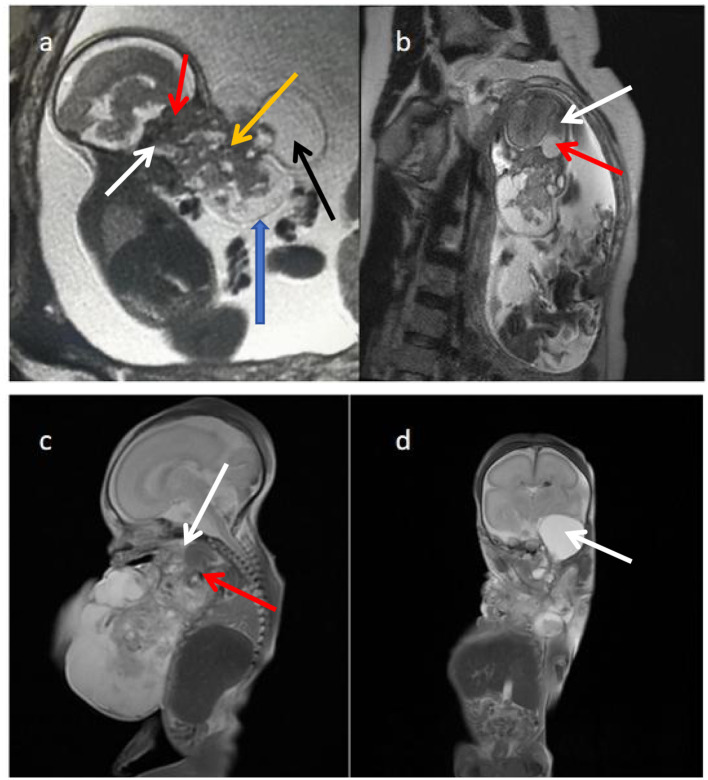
Prenatal and postnatal MRI findings of fetal oral immature teratoma with intracranial abnormality. (a) Prenatal sagittal T2-weighted MRI demonstrates a solid-cystic mass (blue arrows) measuring 6.1 × 6.1 × 6.8 cm arising from the oropharynx with broad attachment to the palate and skull base (red arrows). The solid component (S, orange arrows) shows intermediate signal intensity, while the cystic component (C, black arrows) exhibits markedly high T2 signal. The mass causes severe oropharyngeal compression (white arrows), correlating with the risk of upper airway obstruction. (b) Prenatal coronal T2-weighted MRI reveals a well-defined hyperintense lesion (red arrows) in the right temporal lobe, measuring 17 × 17 mm, compatible with an arachnoid cyst. Mass effect on the adjacent temporal lobe is evident (white arrows). The coexistence of an intracranial cystic lesion with an oral teratoma raises consideration of a developmental field defect involving midline structures. (c) Postnatal sagittal T2-weighted MRI confirms the broad-based attachment of the residual mass to the skull base and mandible (white arrows), with associated bony erosion (red arrows). (d) Postnatal coronal MRI shows a left temporal meningocele (arrow), an additional intracranial finding that further supports the presence of concurrent neurodevelopmental anomalies. S, solid component; C, cystic component.
